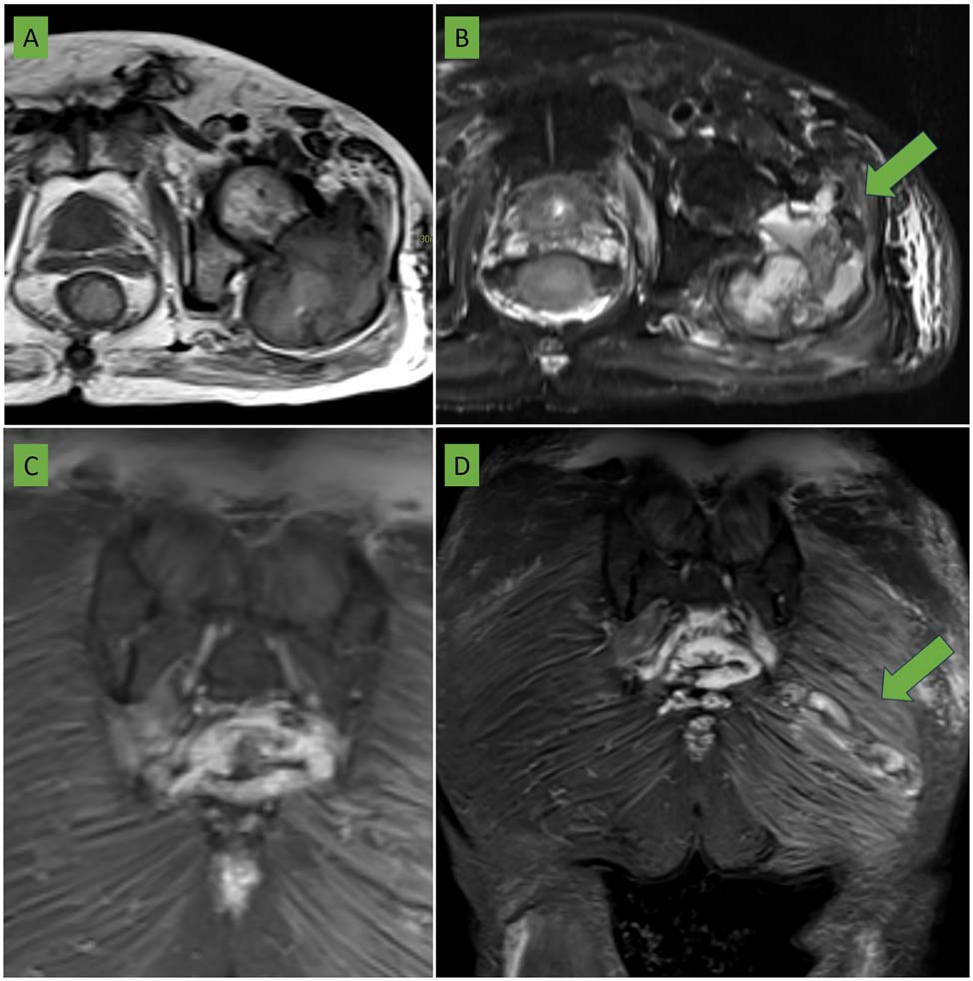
(A) T2 sequence with fat saturation and (B) T1-weighted sequence in axial plane centered on the left hip: a large expansive lesion is visualized centered in the trochanteric region extending to the femoral neck with a heterogeneous matrix of hyperintense predominance with respect to the muscle in both sequences in which cavities with liquid/liquid levels are identified (arrow) compatible with bleeding in different phases. The anterior cortex is focally rotated extending to the medial gluteal musculature (arrow). (C) Coronal section in STIR sequence showing sacrococcygeal involvement of the same characteristics as the femoral lesion. (D) Coronal section in STIR sequence centered in the gluteal region: an elongated lesion with lobulated borders is seen extending from the lateral region of the gluteus maximus to the proximity of the sacrococcygeal region (arrow). It coincides with the biopsy trajectory and is suggestive of tumor seeding.
